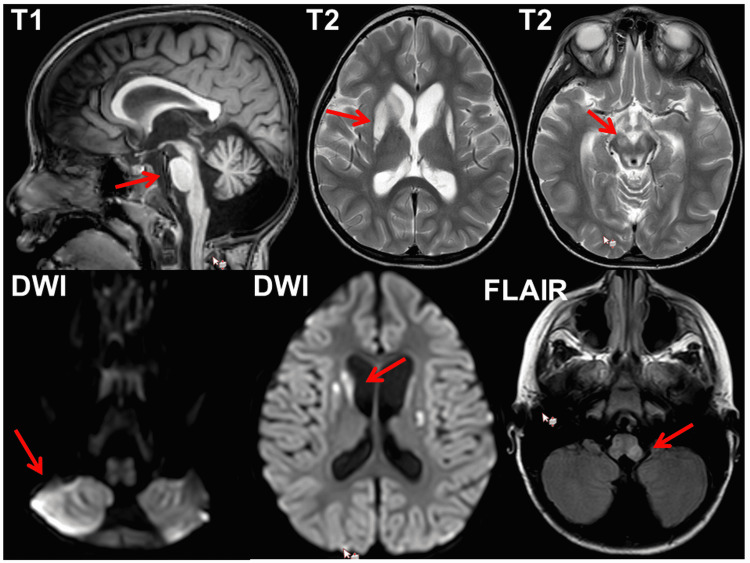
Cerebral MRI of the index patient at the age of 60 months showing cerebellar, pontine, and brainstem atrophy and callosal thinning on T1 (upper left), hyperintense basal ganglia lesions on T2 (upper middle), T2 hyperintense lesions in the midbrain (upper right), DWI hypereintense right cerebellar lesion (lower left), hyperintense basal ganglia lesions on DWI (lower middle), and hyperintense lesions in the medulla on TIRM (lower right) MRI, magnetic resonance imaging; DWI, diffusion-weighted imaging; FLAIR, fluid-attenuated inversion recovery; TIRM, turbo inversion recovery magnitude
